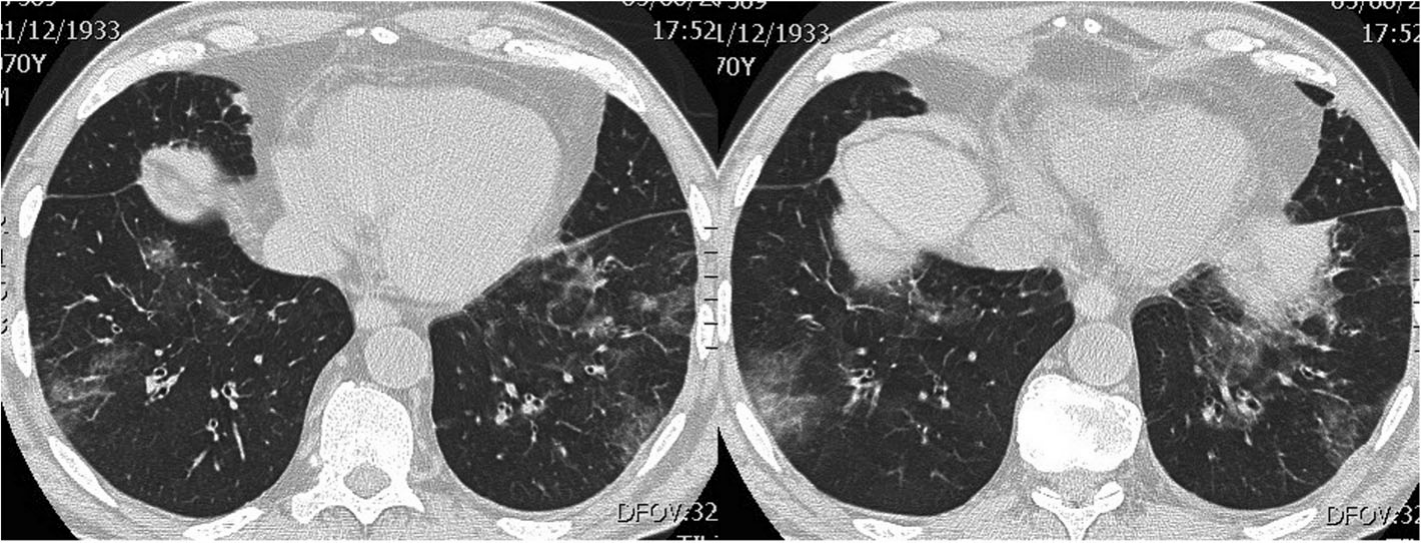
Axial HRCT scan of the chest revealed patchy ground-glass opacities bilaterally, predominantly in the lower lobes of the lungs
